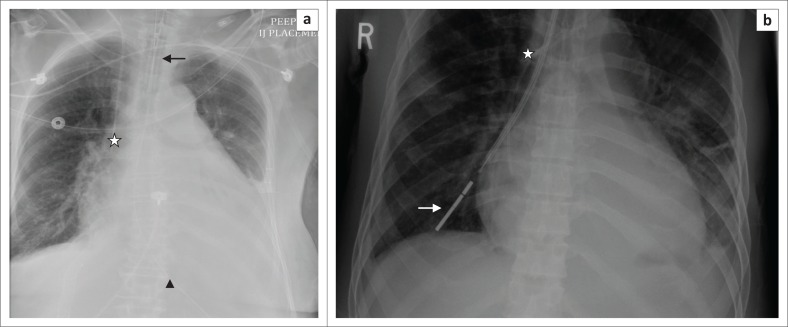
(a) Chest radiograph showing an accurately positioned nasogastric tube with its proximal side hole (arrowhead) and tip located beyond the gastroesophageal junction. In addition, an endotracheal tube (black arrow) and a right internal jugular vein central venous catheter (star) are noted on this chest radiograph; (b) a malpositioned Dobhoff tube (white arrow) in the right main bronchus. Additionally, a left central venous catheter (star) is noted with its tip in the superior vena cava.
